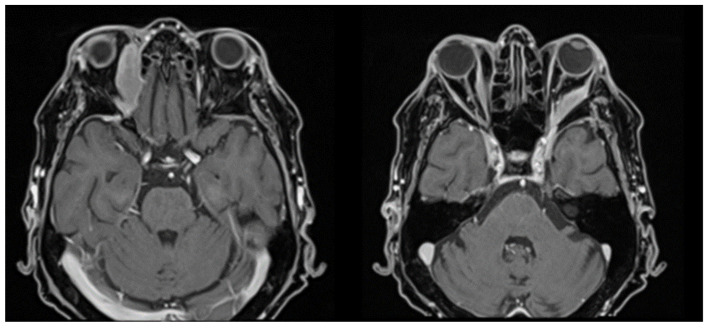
Cerebral MRI—tumoral mass LE.
